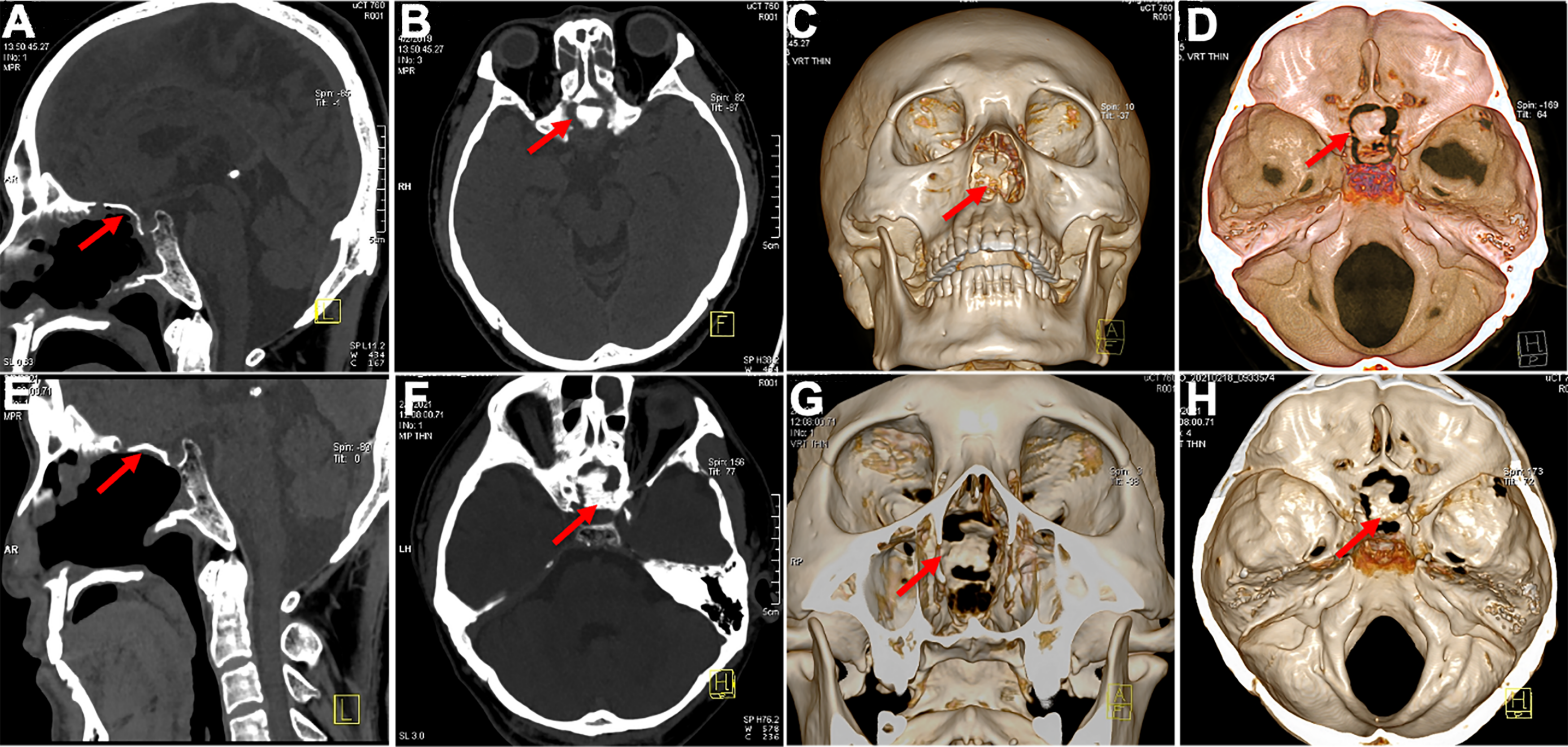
Postoperative bone flap display. (A, B) The bone flap in good position 3 months after surgery (C, D) achieved complete osseous reconstruction (3 months postoperatively). (E, F) CT images showed in situ bone flap 3 years postoperatively and still in the original place. (G, H) Obvious and fresh bone formation around the bone flap 3 years postoperatively.
